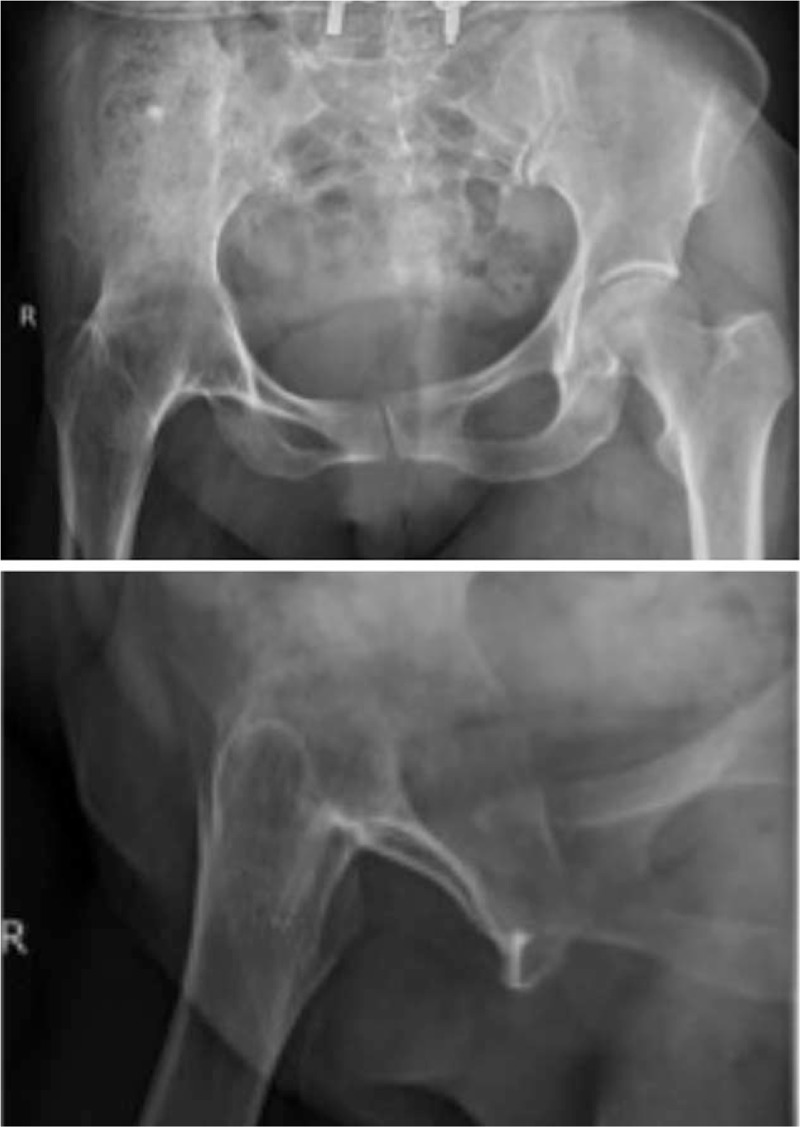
Recent X-ray of right hip joint. Shows the presence of her right hip joint fusion.
